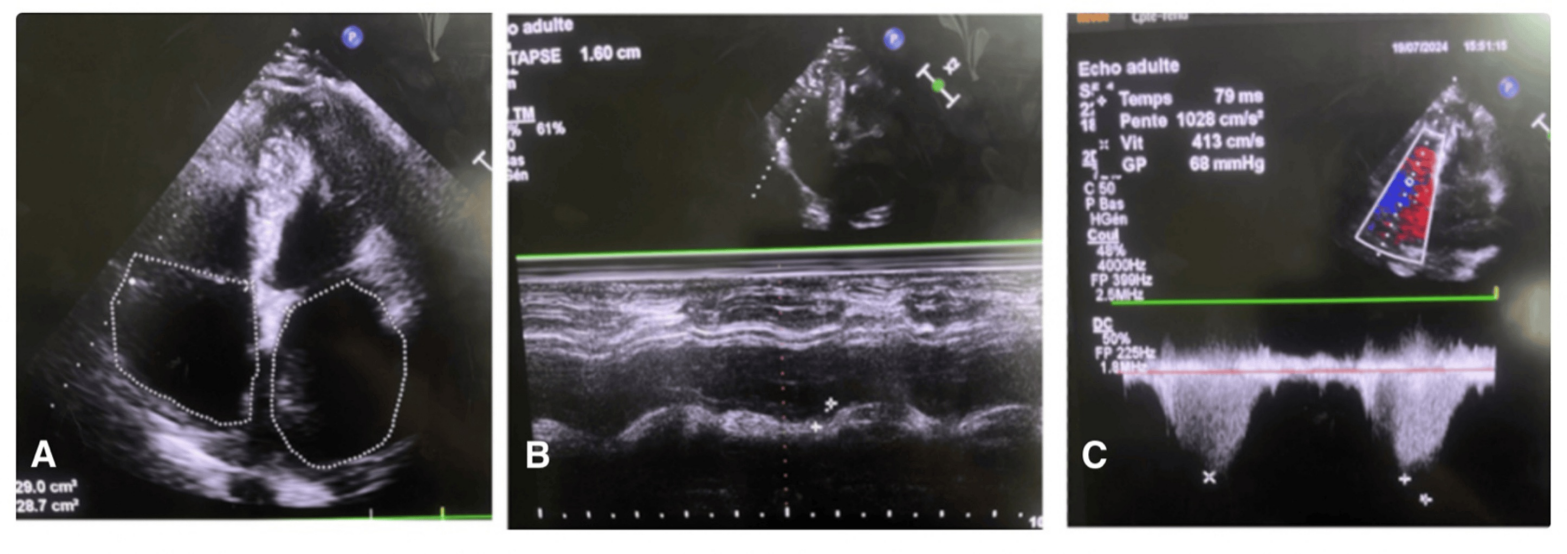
Echocardiographic features. (A) Bilateral atrial dilation. (B) TM-mode demonstrating right ventricular dysfunction. (C) Continuous Doppler assessment demonstrating the presence of significant pulmonary hypertension, with tricuspid regurgitation rated as grade III (Vmax = 4.13 m/s) and an estimated systolic pulmonary artery pressure of 68 + 5 = 73 mmHg.
